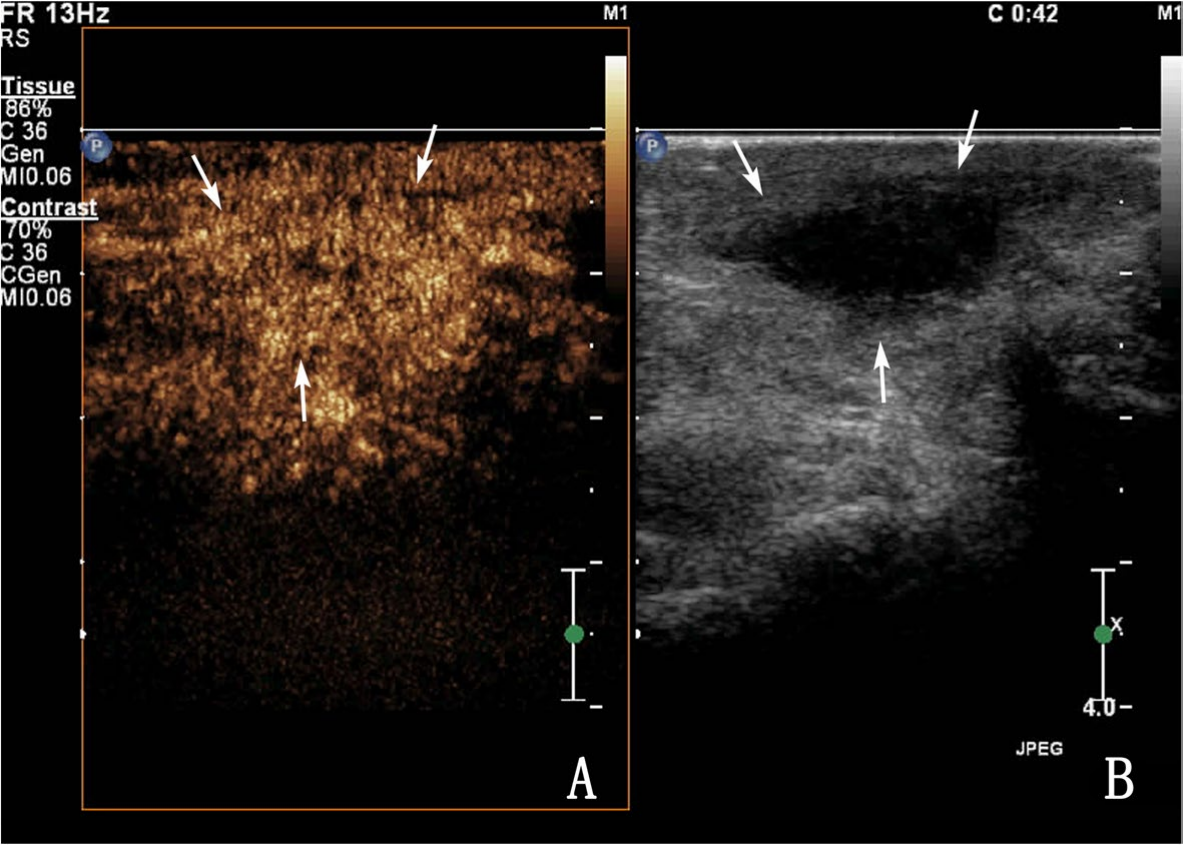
A 43-year-old male with tuberous vas deferens tuberculosis (VD TB). A Routine ultrasonography of a VD TB lesion (white arrow). B Contrast-enhanced ultrasonography of a VD TB lesion showing homogeneous enhancement (white arrow) (type I)
